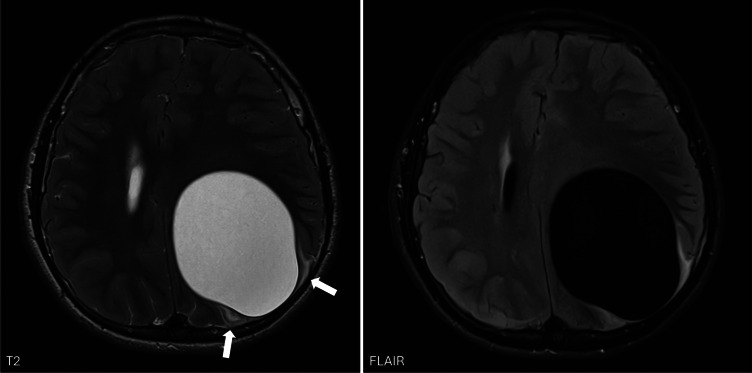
Axial T2‑ and FLAIR‑weighted images show a large intra‑axial cyst in the left frontoparietal region with signal intensity identical to cerebrospinal fluid. There is a significant mass effect with right‑sided midline shift but remarkably little surrounding edema (arrows).
